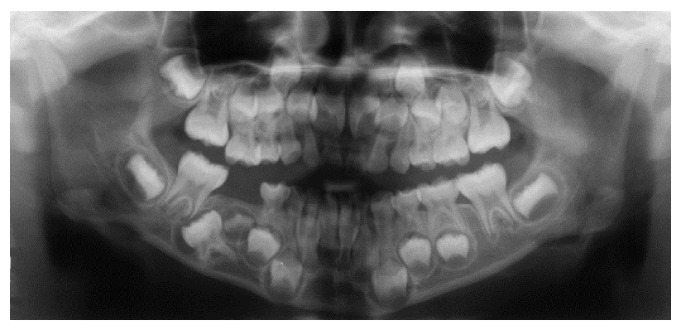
Initial panoramic radiograph: case  2. Complete impaction and distal inclination of the mandibular right second primary molar in the mandibular bone.
